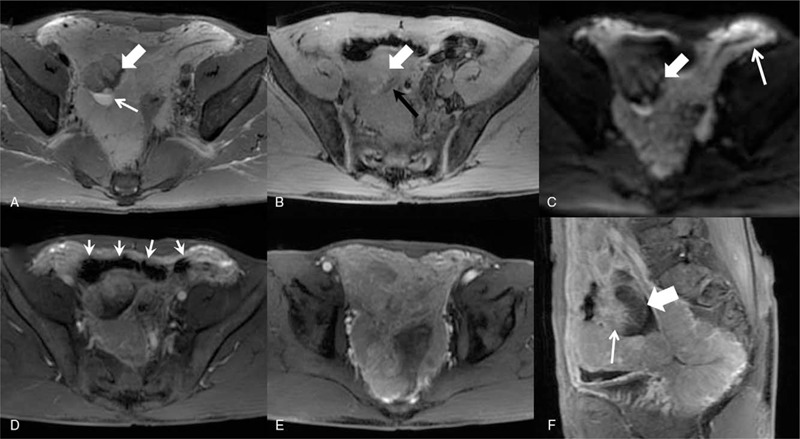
A: axial view of T2WI showed a pelvic hyperintense lesion with hypointense bleeding area (thick arrow) and capsule, as well as focal cystic change (thin arrow); B: axial view of T1WI revealed hyperintense bleeding area (thick arrow) within the pelvic lesion and hypointense capsule (thin arrow) surrounding the lesion; C: axial view of DWI showed pelvic lesion with mixed signal (thick arrow) and greater omental lesion with restricted diffusion (thin arrow); D: axial view of contrast-enhanced MRI showed a clear boundary between the lesion and non-enhance hypointense small intestine (thin arrow); E: significantly enhanced pelvic and greater omental lesion; F: sagittal view revealed the pelvic necrosis area (thick arrow) and enhanced area (thin arrow).
